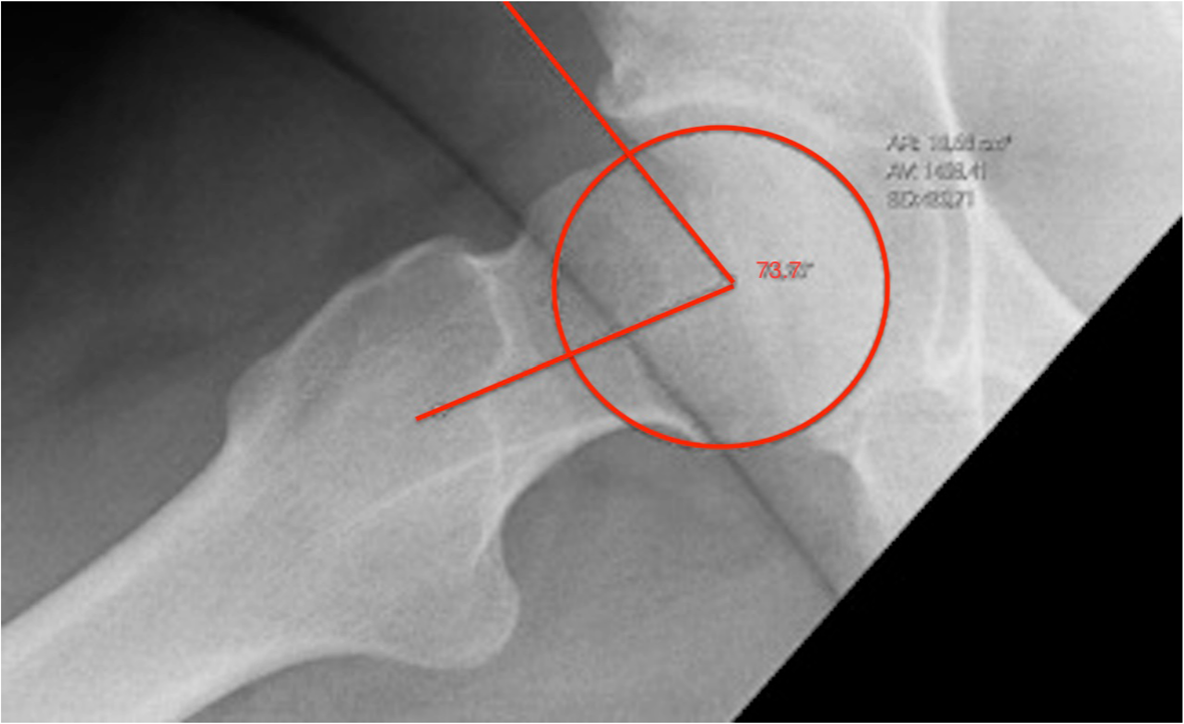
example of an alpha angle measured on a Lauenstein x-ray
